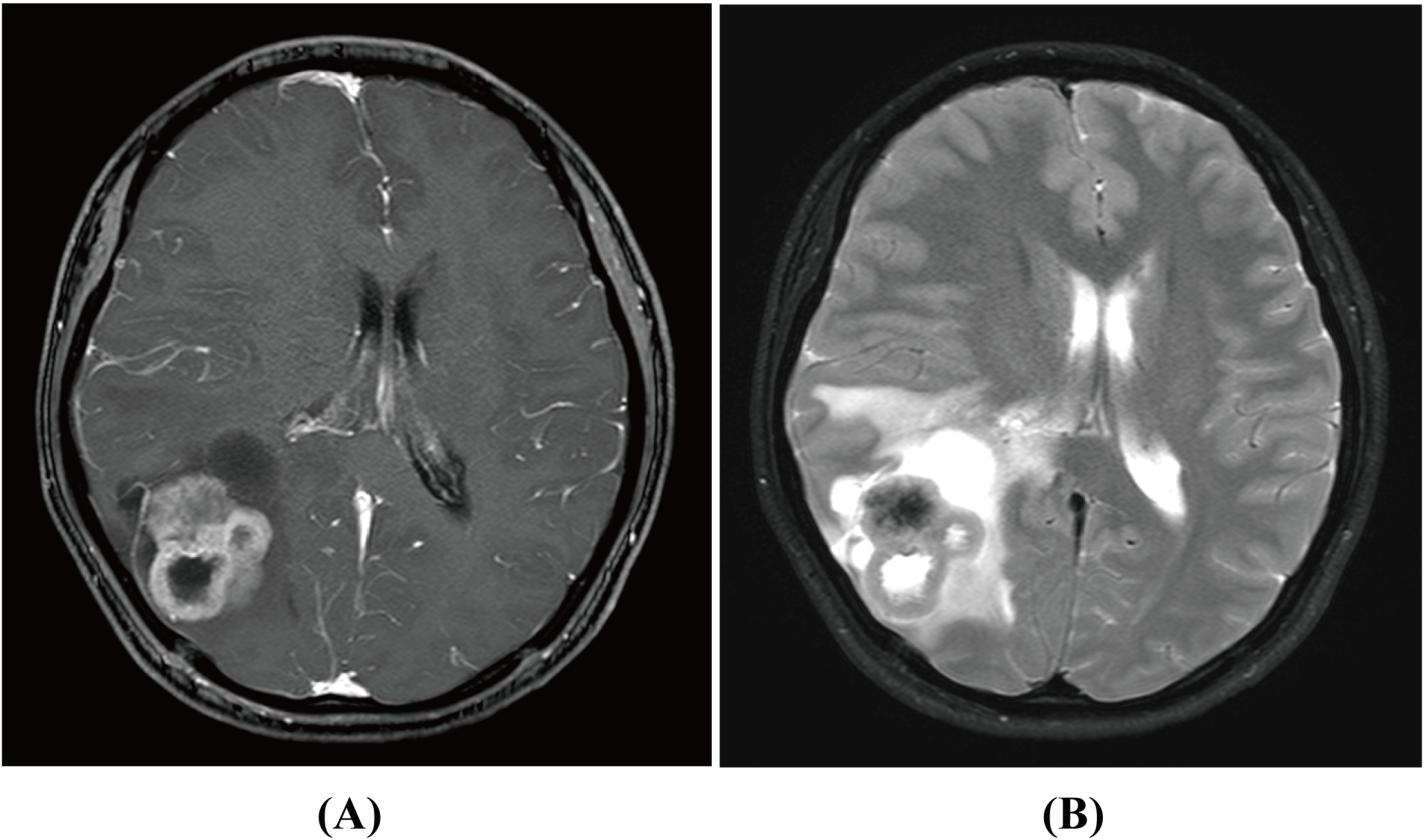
Magnetic resonance imaging (MRI) scan of primary intracranial myofibroblastic sarcoma in contrast-enhanced T1WI (A) and T2WI (B) before surgery.
